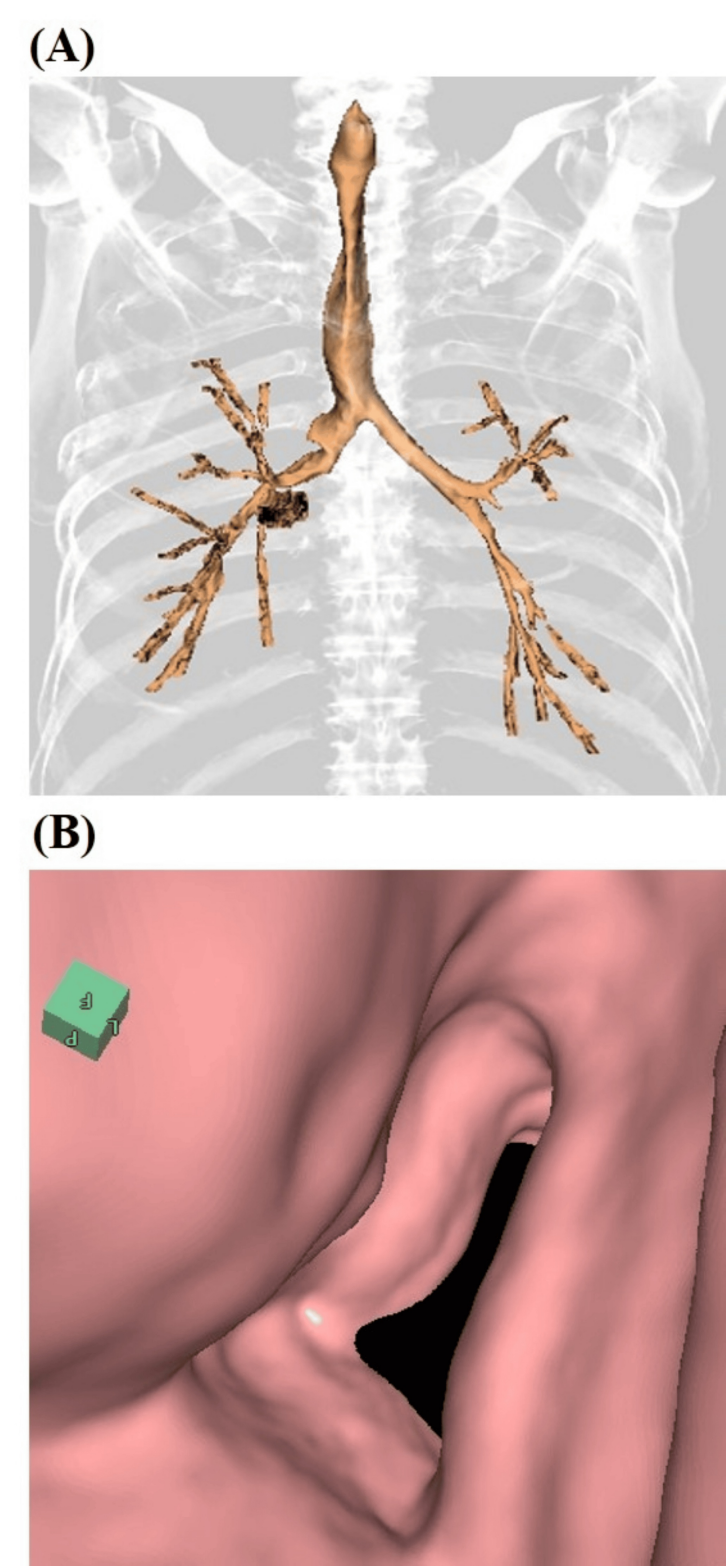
Coronal (A) and virtual (B) bronchoscopy images of the saber-sheath trachea on a dimensional chest computed tomography reconstruction image.
